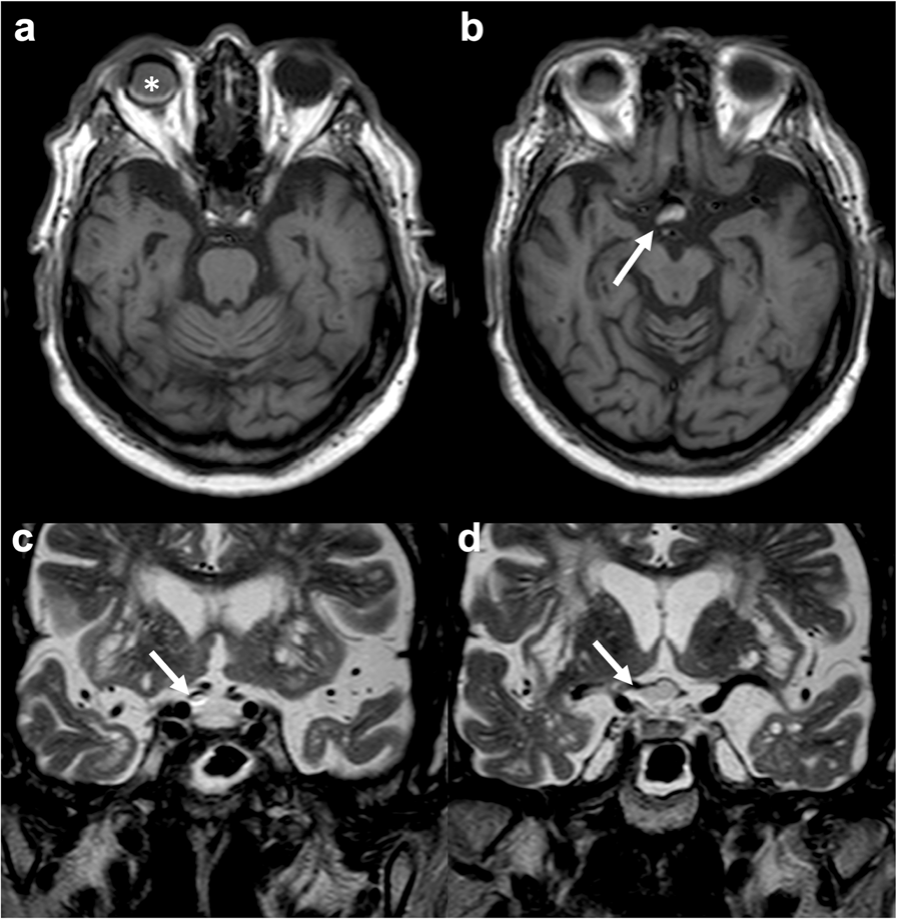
Silicon oil migration. Axial T1-weighted (a, b) and coronal T2-weighted (c, d) images demonstrating migration of silicon oil used to treat right retinal detachment (asterisk in a) through the right optic nerve sheath up to the right half of the optic chiasm (arrow in b, c, and d). The migrated material has heterogeneous signal also due to chemical shift artifact
